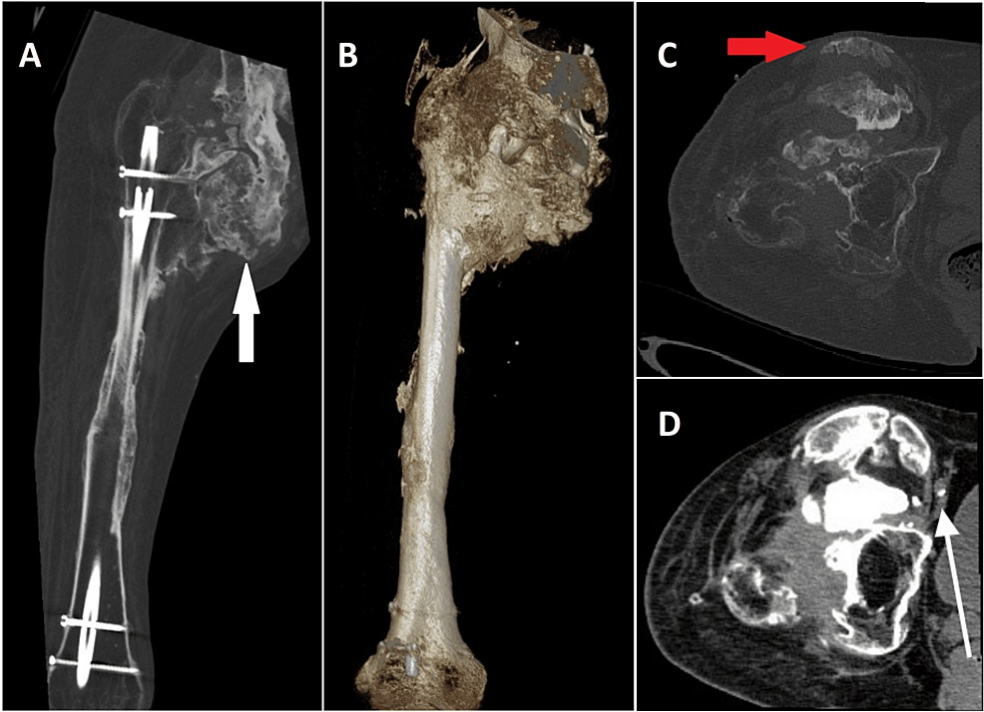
A) Coronal CT of the femur showing the bony bridges (white arrow) between the proximal femur and the right hemi-pelvis, B) 3D reconstruction of the hip ankylosis, C) transversal CT showing the proximity of the anterior calcifications to the skin (red arrow), and D) transversal CT showing the femoral nerve and vessels completely embedded in the NHO (white small arrow). 3D, three-dimensional; NHO, neurogenic heterotopic ossification
